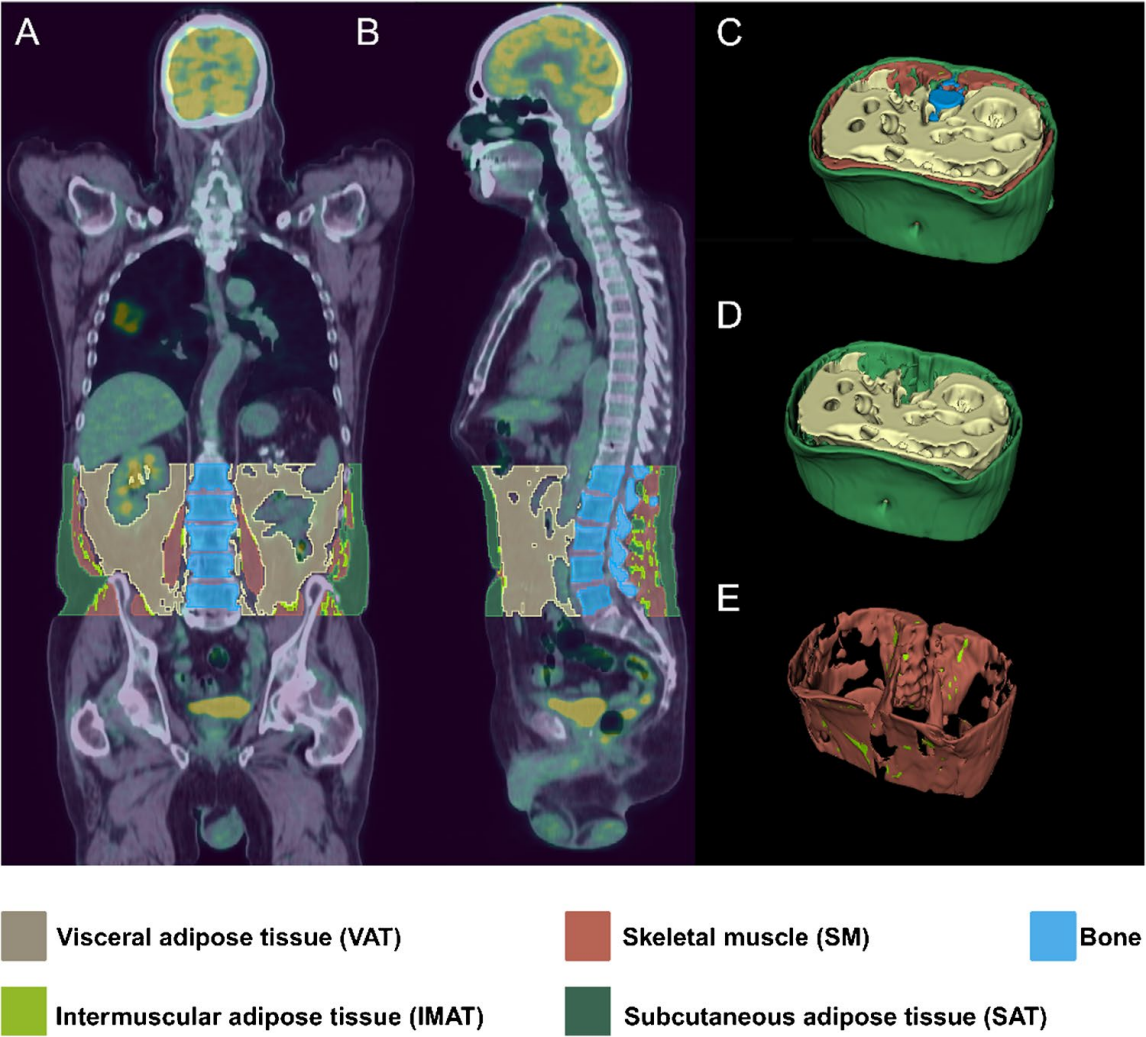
Automated body composition segmentation in NSCLC patients using ^18^F-FDG-PET/CT imaging. An example of automated segmentation of body compositions in an NSCLC patient is depicted using ^18^F-FDG-PET/CT images in the sagittal (A) and coronal (B) views. (C) A 3D rendering of all previously mentioned tissues, including visceral adipose tissue (VAT), subcutaneous adipose tissue (SAT), skeletal muscle (SM), and intermuscular adipose tissue (IMAT). (D) A 3D rendering of IMAT and SM. (E) A 3D rendering of SAT and VAT. The different colors represent the individual tissue classes: khaki for VAT, red for SM, blue for bone, green for IMAT, and bottle green for SAT
